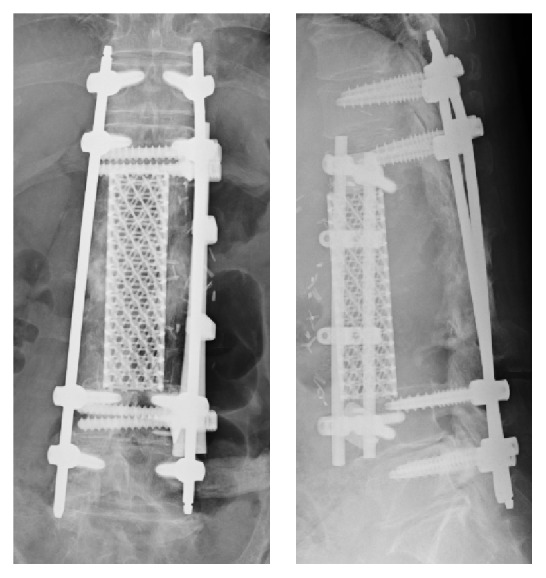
X-ray image of the spine after surgery.
